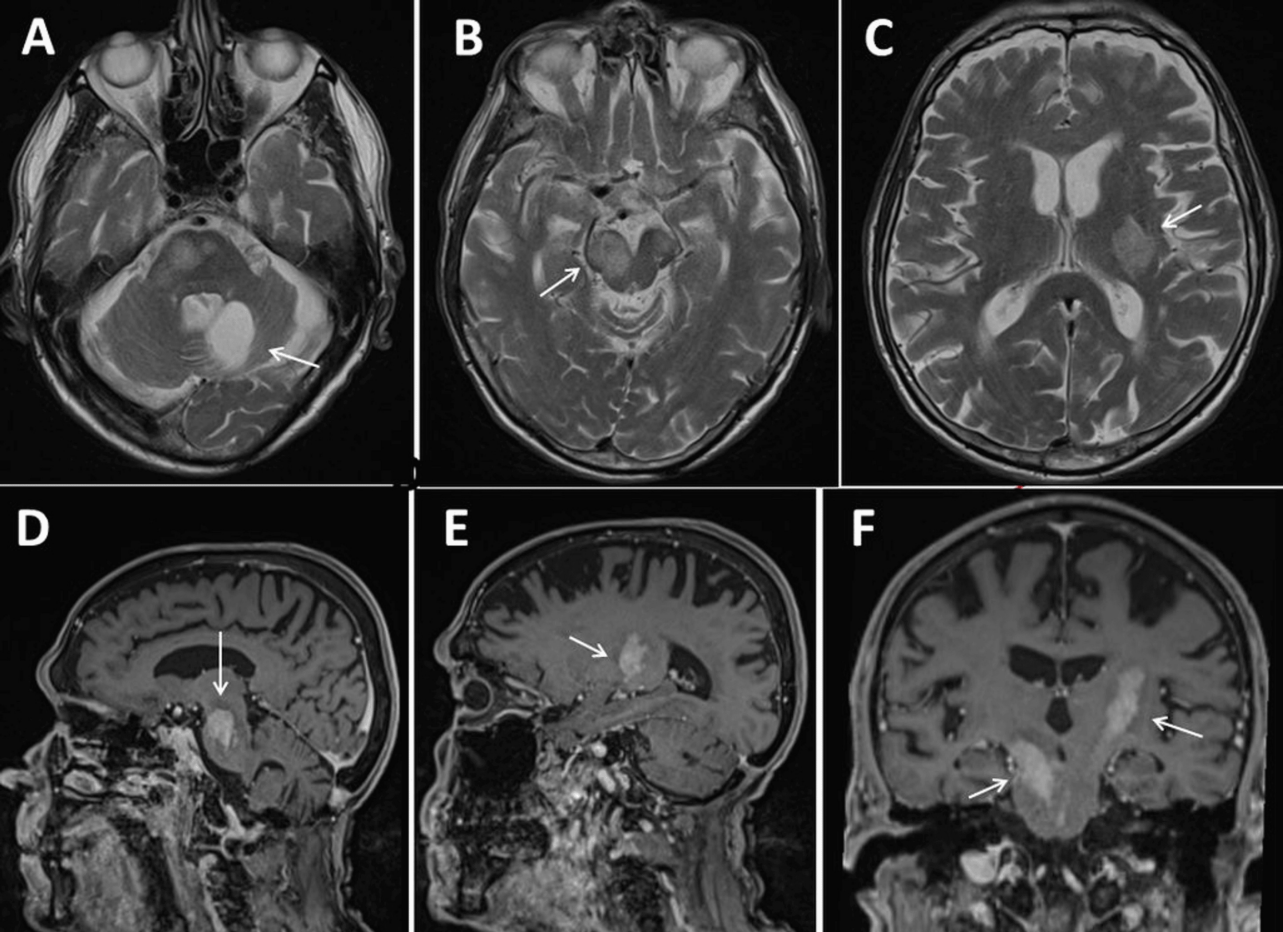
Cerebral MRI at 86 years of age showing the old surgical defect in the left cerebellum after resection of the lymphoma (A). Cerebral recurrence of PCNSL in the predunculi cerebri (B), the left basal ganglia (C) on axial images, on sagittal images (D, E), and on coronary images (F). PCNSL: Primary central nervous system lymphoma
